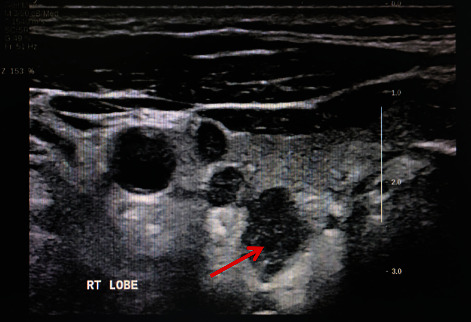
This is the thyroid ultrasound of patient 5 who underwent endoscopic surgery 8 months after the initial surgery. The ultrasound showed a local recurrence in the right lobe of the thyroid, with unclear margins and irregular shape.
